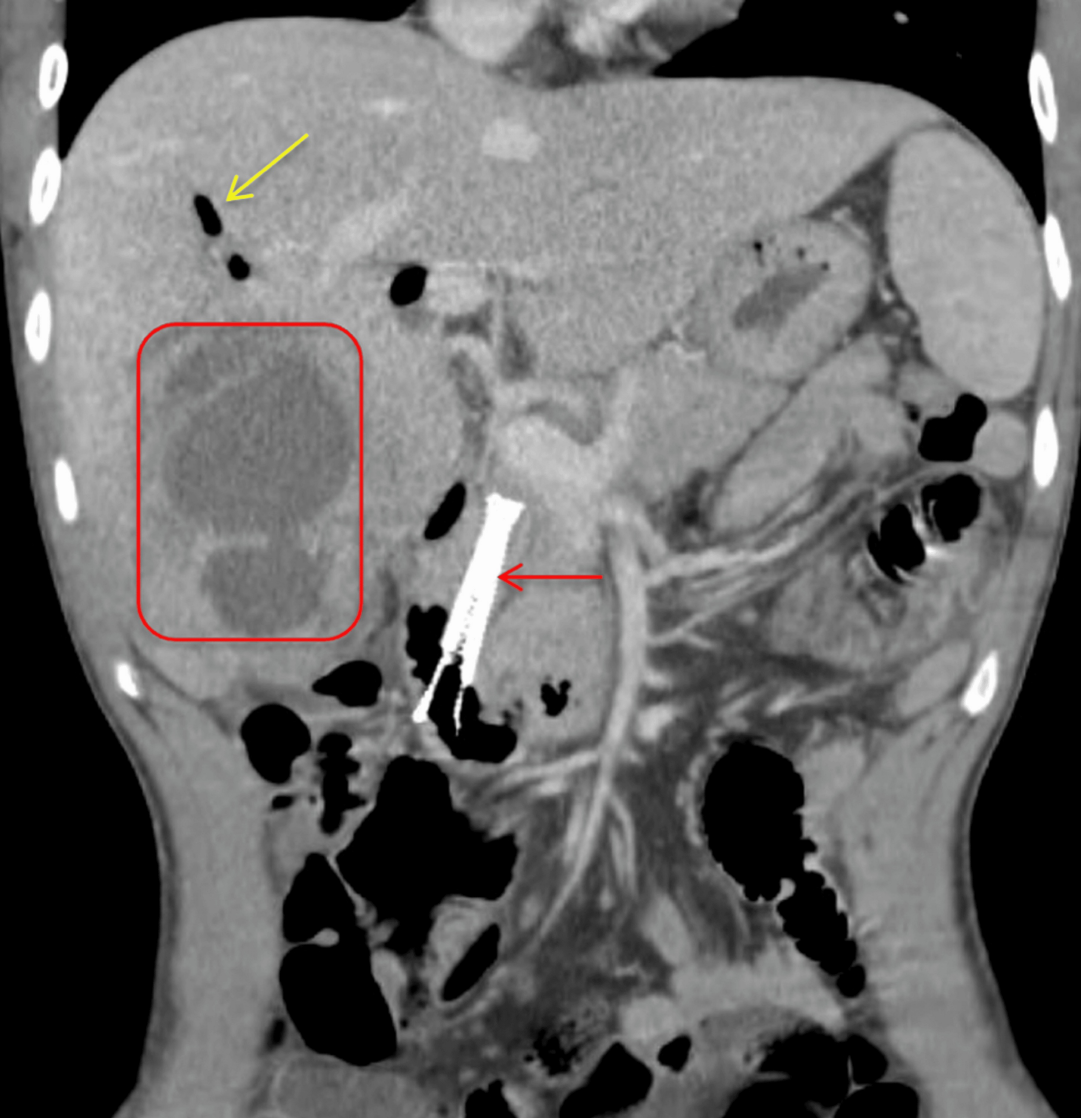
CT abdomen and pelvis with IV contrast (coronal view) demonstrating a peripheral enhancing fluid collection in the right hepatic lobe (red rectangle). Mildly distended intrahepatic biliary ducts are visible with air visible in the biliary tree (yellow arrow), likely related to the common bile duct stent extending into the duodenum (red arrow).
